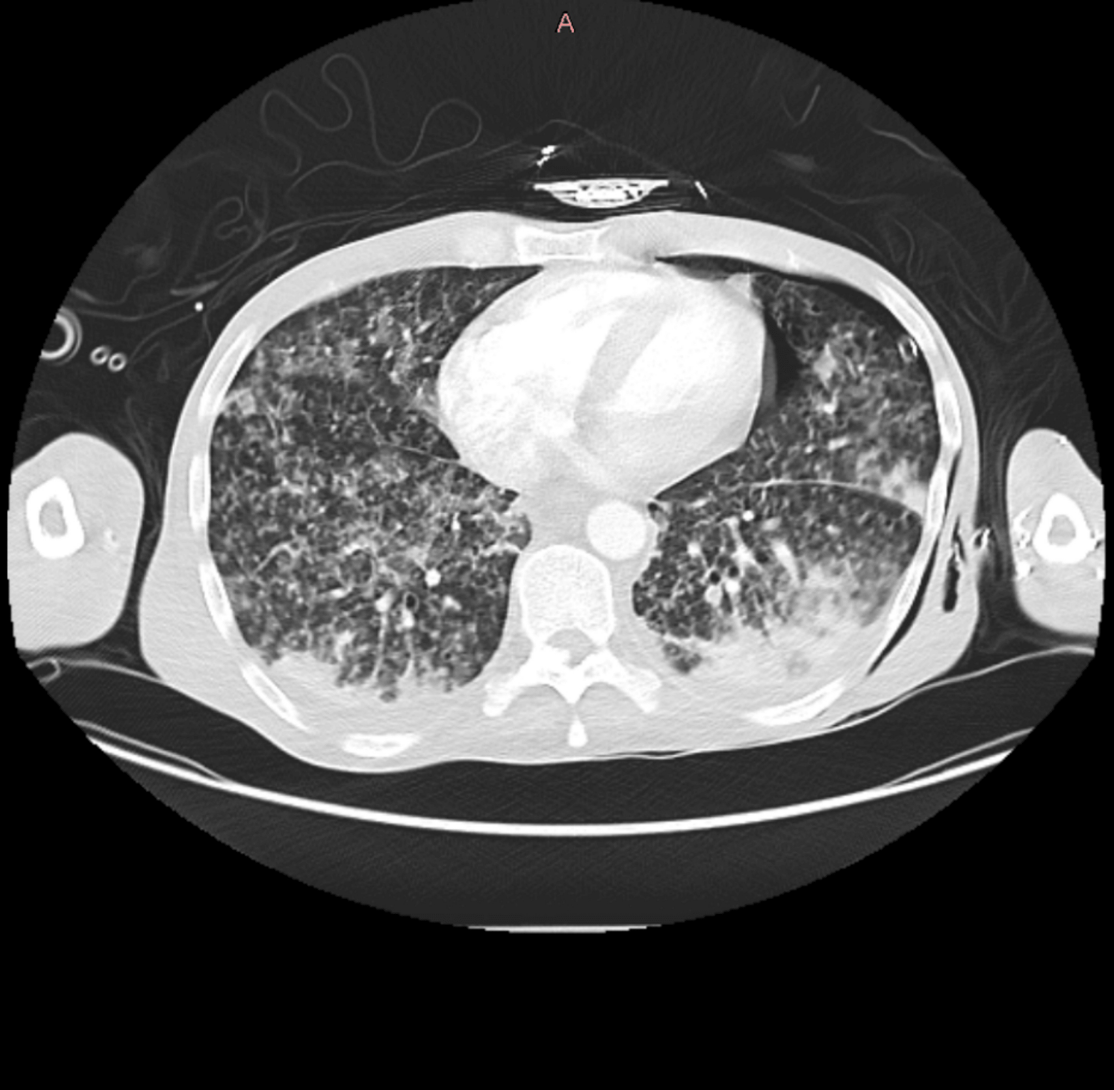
Repeat CT thorax done (08/03/2024) showing disease progression
